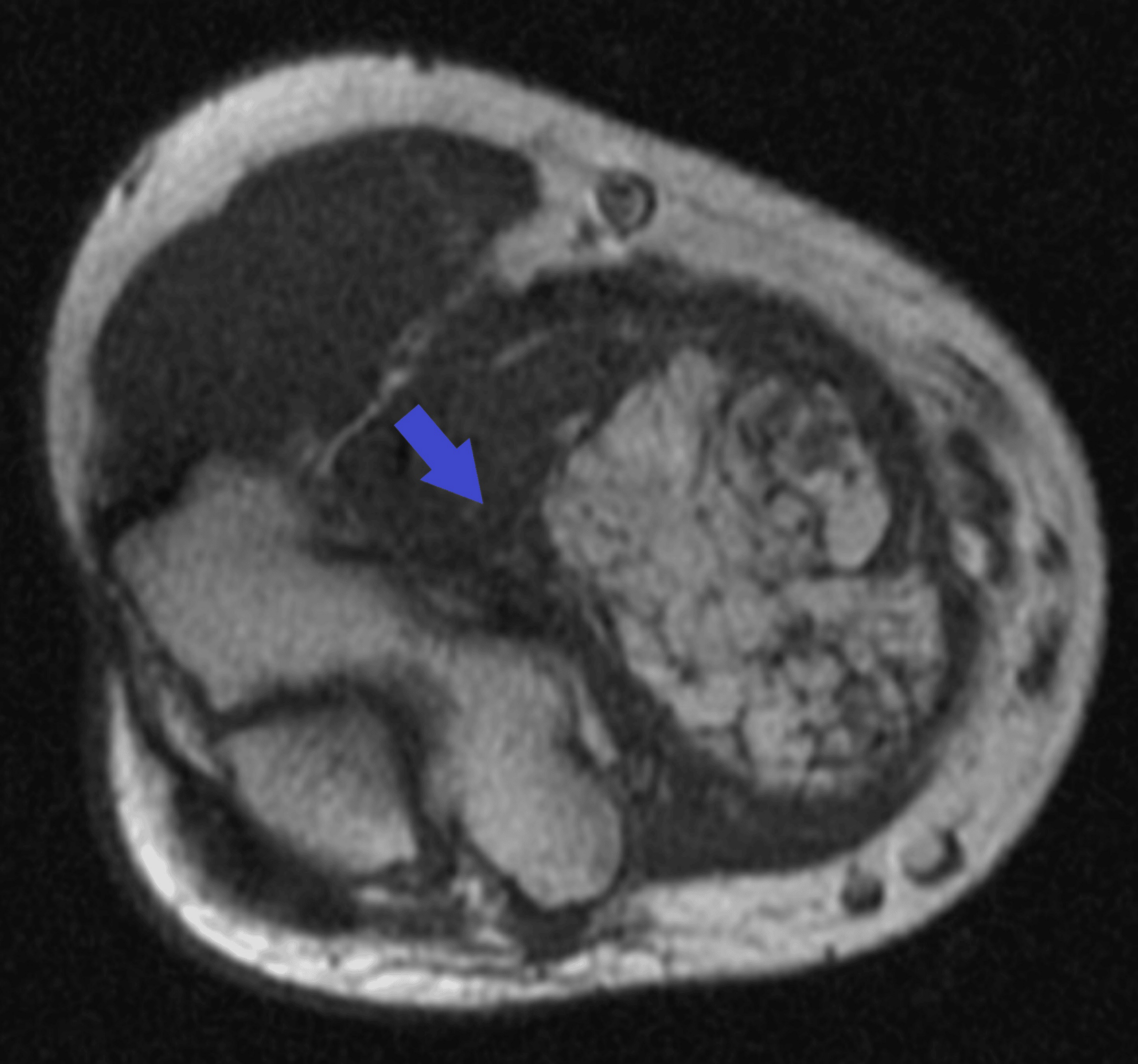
T2 weighted MRI image of right upper extremity showing a heterogeneous soft tissue with bone abutment (blue arrow)
